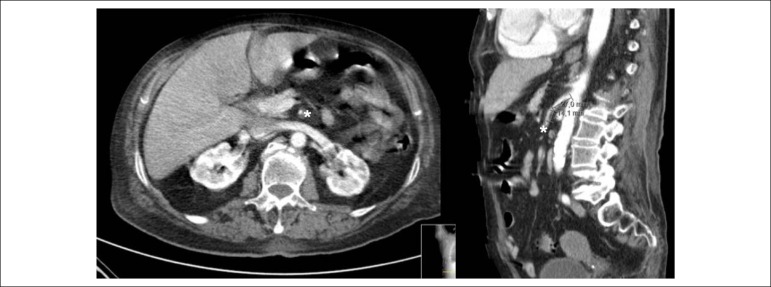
Abdominal computed tomography showing a thrombus in the superior mesenteric artery (white asterisk).
